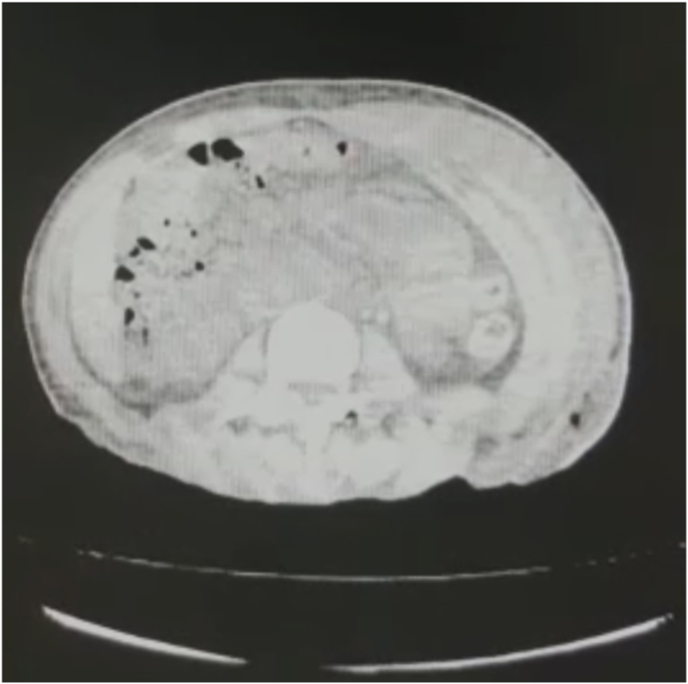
Computed tomography showing gas and edema in subcutaneous and deep fascial plains of back and abdomen.
